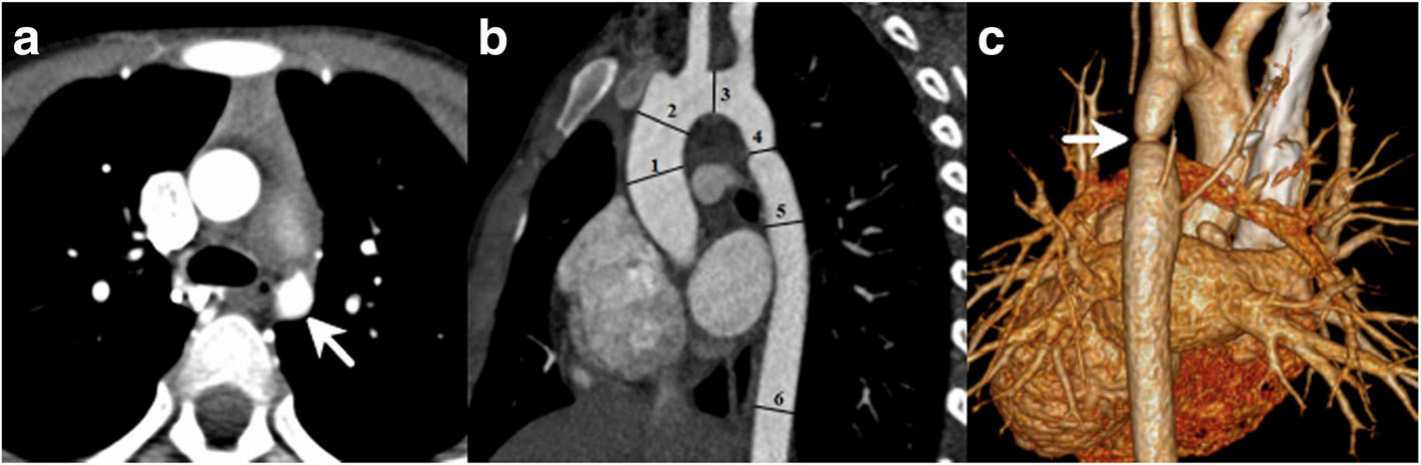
CT images of a 10-year-old man with coarctation of the aorta. a Axial image shows the significant stenosis of aortic isthmus (white arrow). b Sagittal multiplanar reformatted image shows the measurement of aortic diameters at different levels: 1, ascending aorta; 2, pre-coarctation aorta; 3, aortic arch; 4, site of coarctation; 5, descending aorta after the site of coarctation; 6, descending aorta at level of diaphragm. c Volume rendering image shows a short segment aortic narrowing (white arrow) below the left subclavian artery
